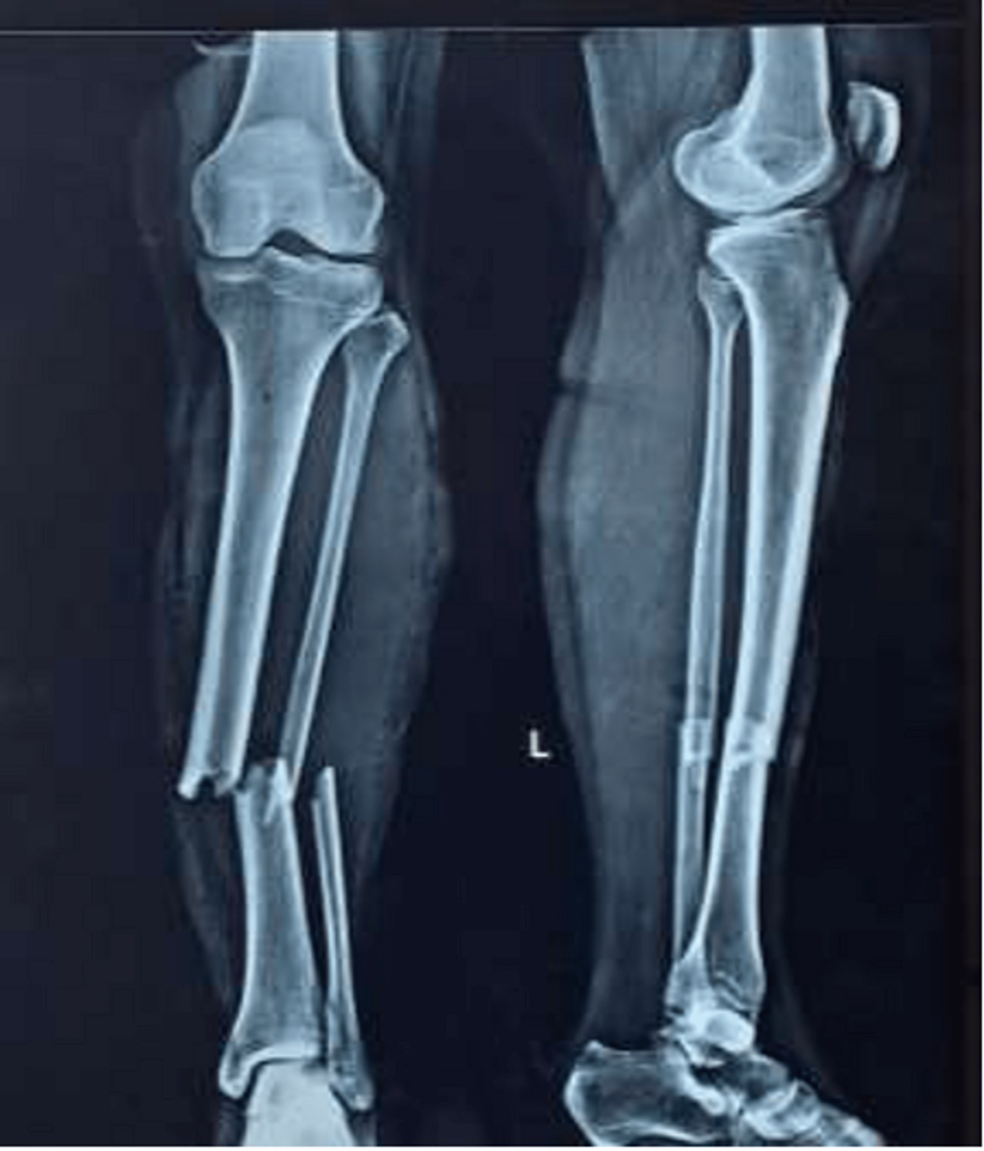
Preoperative radiograph of the leg
